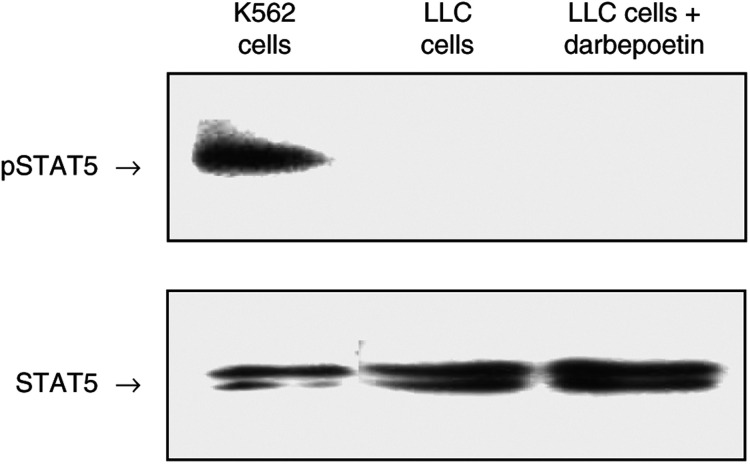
Lewis lung carcinoma cells were serum-starved for 5 h and then stimulated for 20 min with darbepoetin alfa (250 ng ml^−1^), or left untreated as a control. K562 cells, in which STAT5 is constitutively phosphorylated, were used as a positive control. After treatment, the cells were washed and lysed. Following separation of proteins by SDS–PAGE, blots were probed with anti-phospho-specific STAT5 antibody and pSTAT5 expression was analysed by X-ray autoradiography. Data represent typical results of one of three independent experiments. The membrane was stripped and probed with an antibody that recognises total STAT5 (bottom).
